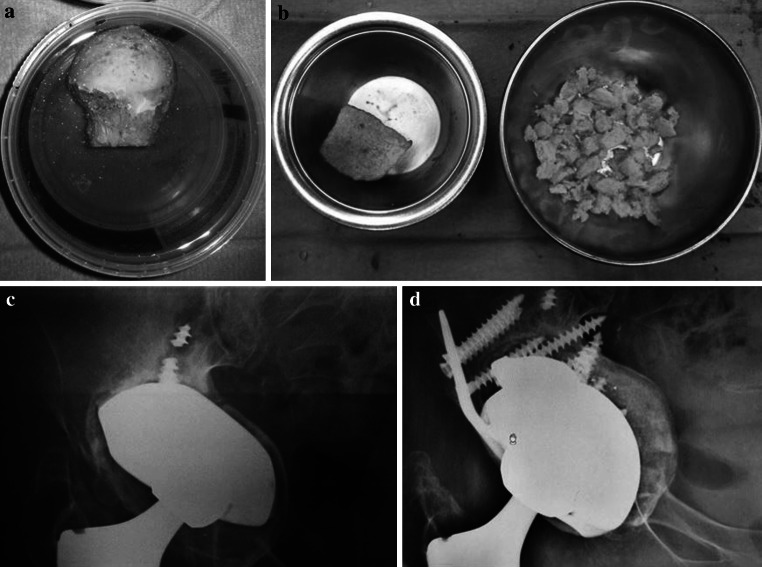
a, b Morcelized homologous bone graft obtained from a banked femoral head. c Severe acetabular bone loss in a mobilized hip revision cup. d X-ray control at 2 years with evidence of bony stable osseointegration of the new cup in the remodeled bone graft
